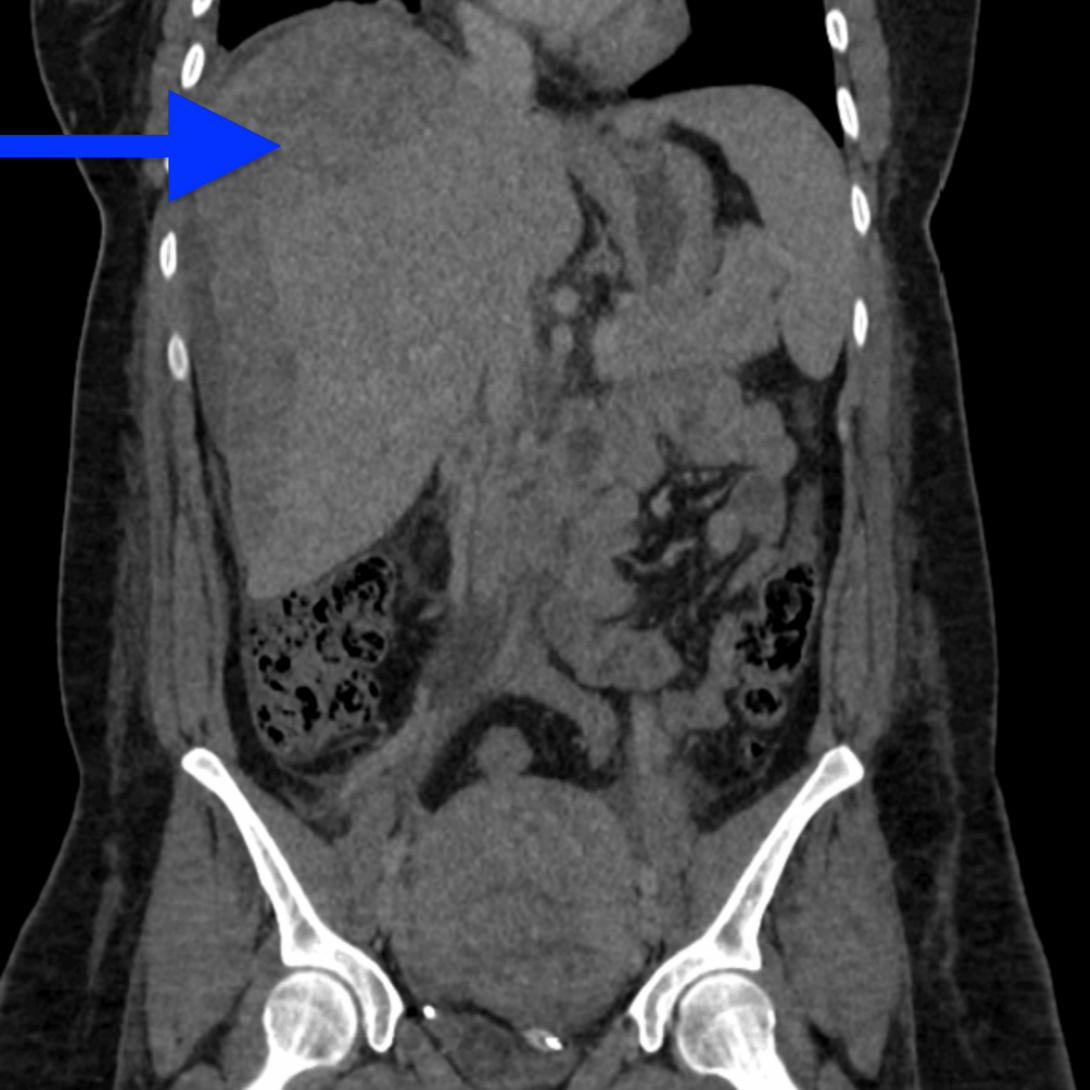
Coronal view of the CT performed on day one, showing a large subcapsular hepatic haematoma (blue arrow)
